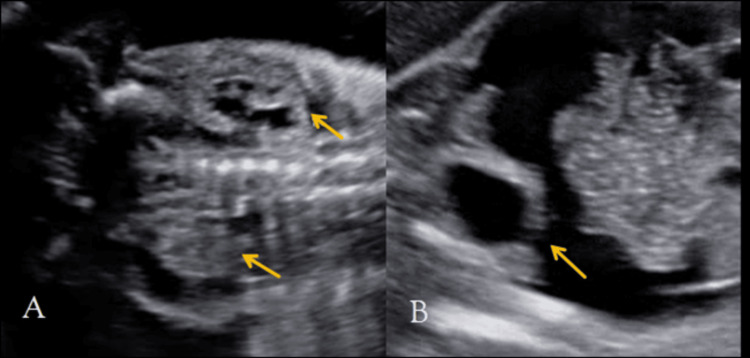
Ultrasound of the upper urinary tract shows bilateral hydronephrosis (arrows, A). A breech in the continuity of the urinary bladder wall is seen (arrow, B), indicating the cause of ascites in a fetus with a posterior urethral valve.
